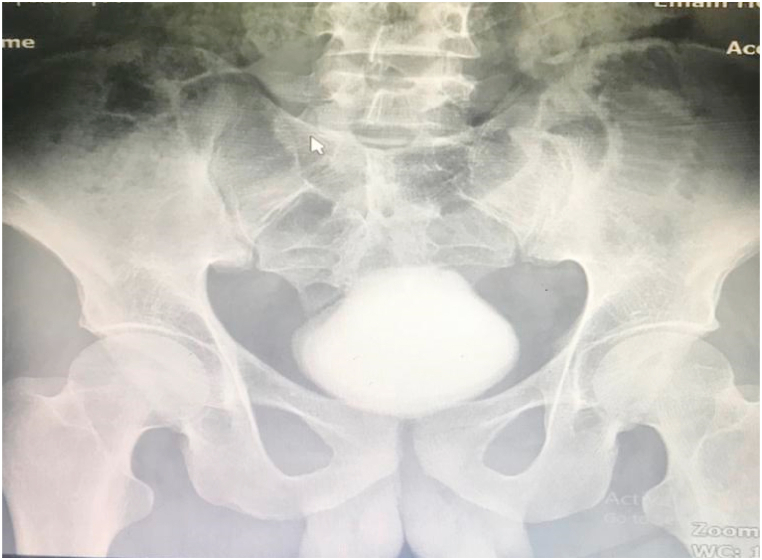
Abdomen X-ray of the patient, showed a large urinary calculus in the bladder.
